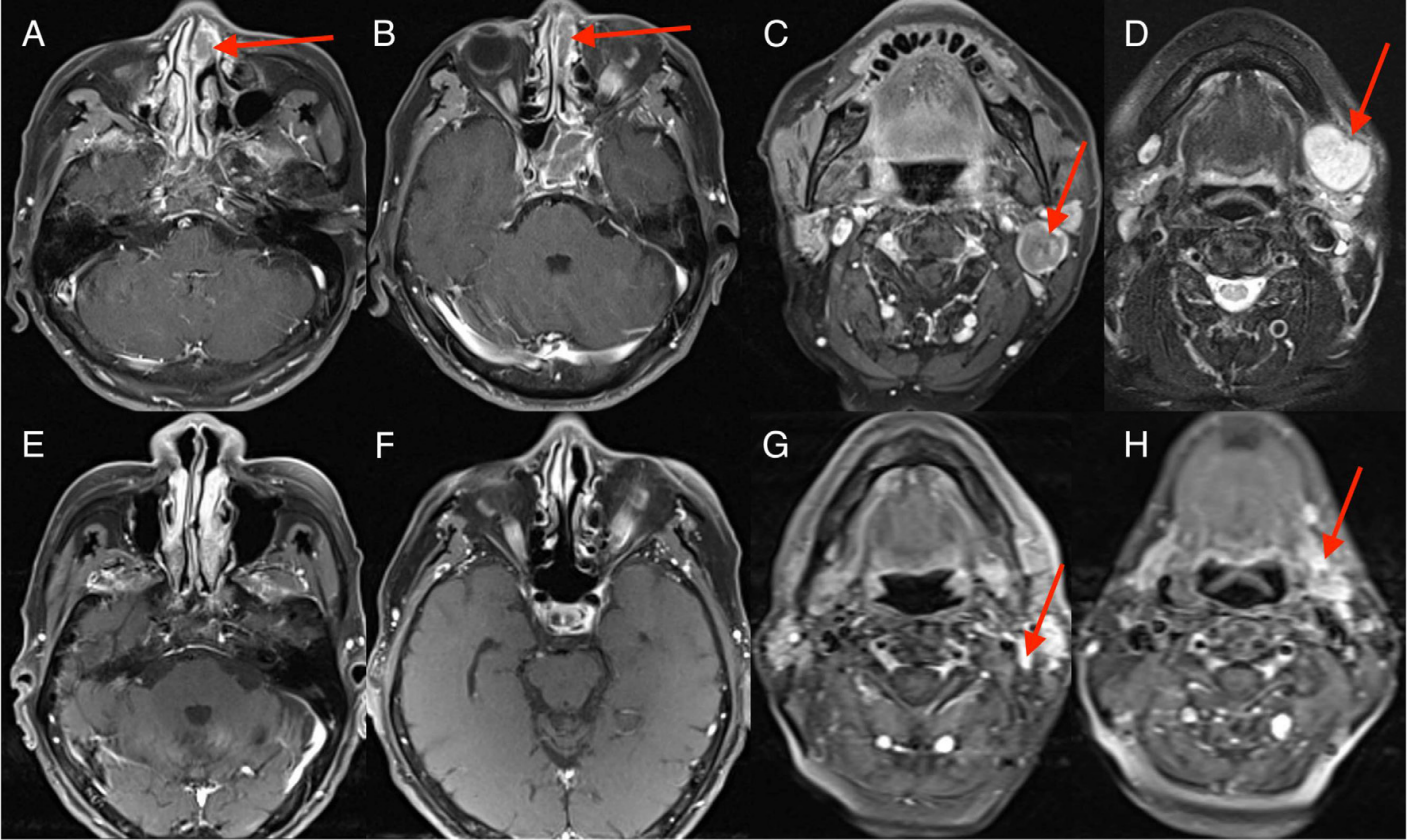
Imaging changes during treatment. Magnetic resonance imaging (MRI) images demonstrating diagnosis, treatment responses. [(A–D) Sinonasal squamous cell carcinoma (SCC) was first diagnosed (15 July 2021); MRI scan showed a 3.7 cm × 2.0 cm soft tissue lesion in sinonasal and enlarged lymph node in the left upper neck region. (E–H) reduced tumor during four cycles of EP and durvalumab (October 15, 2021); MRI scan showed sinonasal SCC disappeared and neck lymph nodes reduced.].
